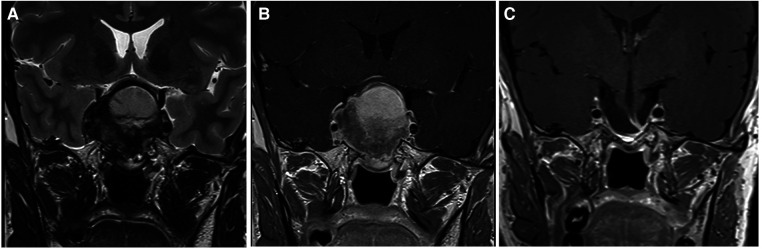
This large pituitary adenoma was classified as Knosp 3a and grade 1 according to the relationship with the diaphragm (Pictures A and B showing a coronal T2- and T1-weighted MRI after gadolinium administration, respectively). The diaphragm was wide open and the adenomas presented an oval shape. A gross total resection was possible through a classic endoscopic endonasal approach (Picture C), and no recurrent tumor is evident at 2 years of follow-up.
